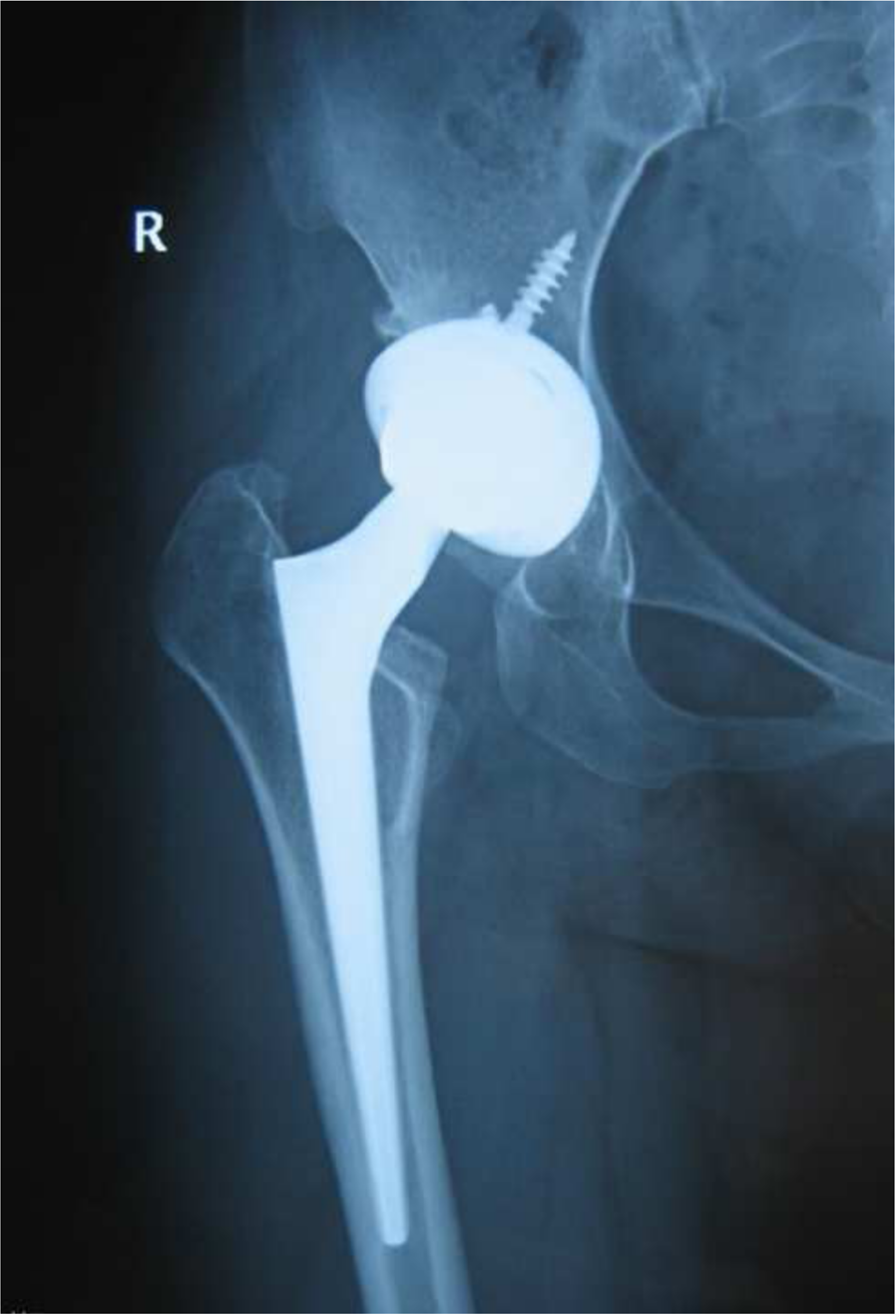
Radiographic aspect in the anteroposterior view at 8 years of follow-up: the implant is stable, and no signs of bone resorption are noticeable
